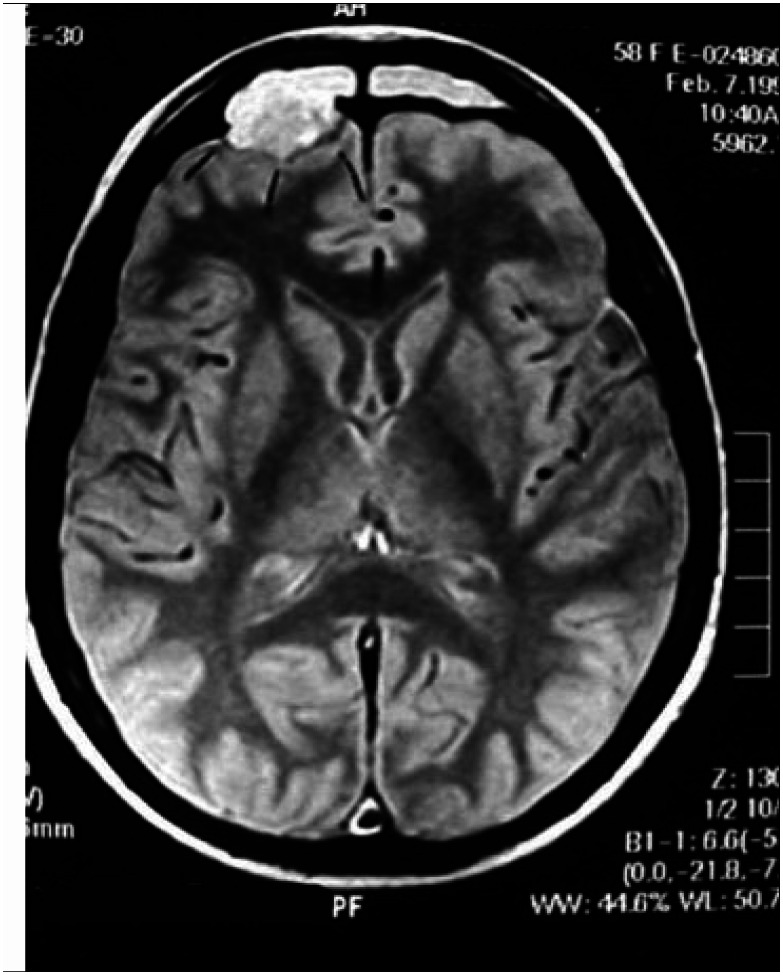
MRI demonstrated dural thickening in communication with the frontal mass.
